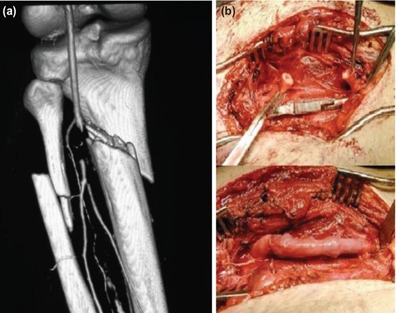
(a) CT Angiogram showing Injury to tibio-peroneal trunk in Grade 3C open fracture (b) managed by thrombectomy followed by reverse saphenous vein graft.
